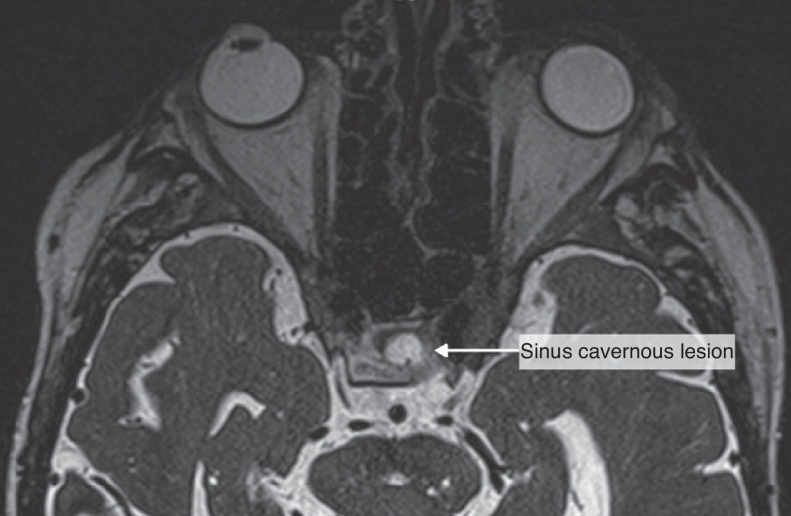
The sinus cavernous lesion (MRI, T2).
